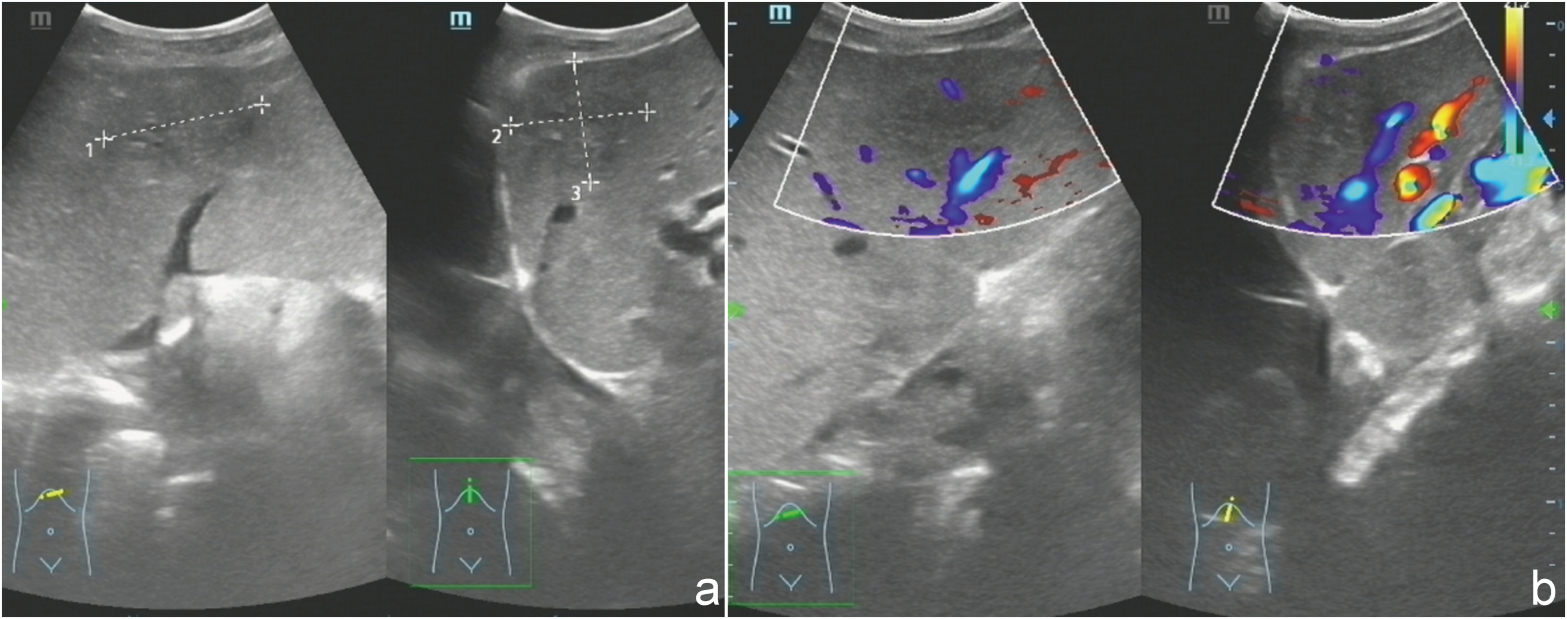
(a) Ultrasonography demonstrated a heterogeneous, patchy hypoechoic lesion with poorly defined margins located in segment S2 of the liver. (b) Color Doppler flow imaging (CDFI) revealed scattered intralesional and perilesional vascular signals.
